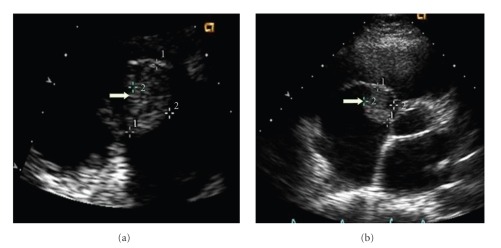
This is a transthoracic echocardiogram. White arrows point to the 2.6 × 1.7 cm pedunculated vegetation.
